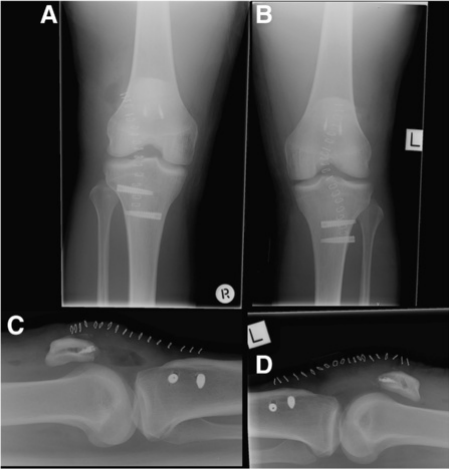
Post-operative Xrays demonstrating patellar and tibial tunnel placement. a AP view right knee, (b) AP view left knee, (c) Lateral view right knee and (d) lateral view left knee. These tunnels demonstrate the way in which the ‘figure 8’ reconstruction was performed
